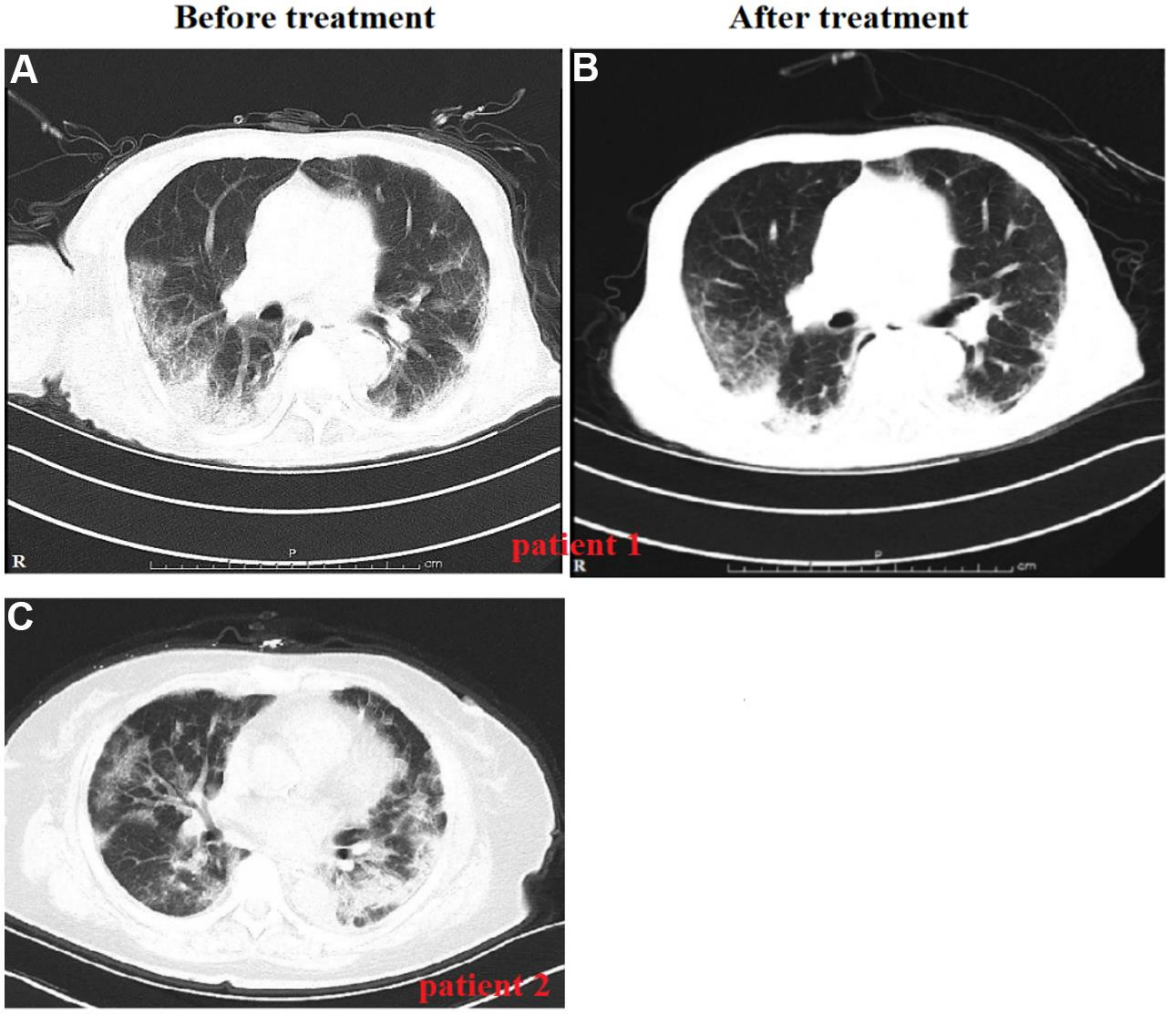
Variation in radiological findings in patients with severe and critical COVID-19 during disease course. Chest computed tomography (CT) shows severe multiple patchy shadows in both lungs on day 20 of symptom onset (examined upon admission) before treatment (A). Chest CT shows improved patchy shadows on day 39 of symptom onset for the same patient after treatment (B). Chest CT for a 68-year-old female who presented with high fever and dyspnea before treatment (C). Followed-up CT assessment was not performed because the patient died of her severe illness.
